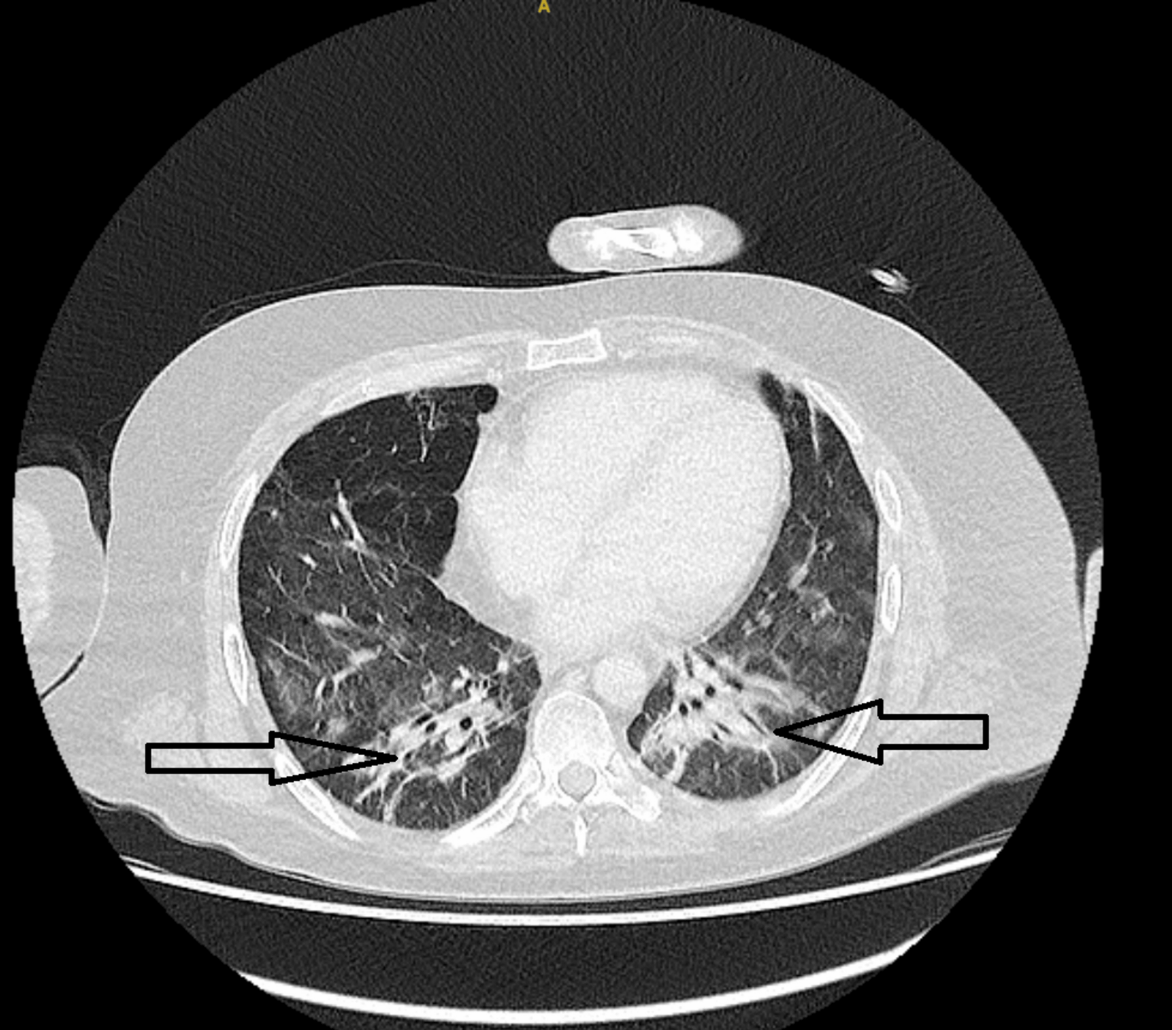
CT chest (Sept 2022, non-contrast) shows interval improvement in pulmonary findings after corticosteroid therapy, with decreased bilateral ground-glass opacities and consolidations. Black arrows indicate residual fibrotic bands and resolving infiltrates, mainly in the lower lobes, reflecting partial resolution of HLH-associated pulmonary changes. NCCT: non-contrast computed tomography
